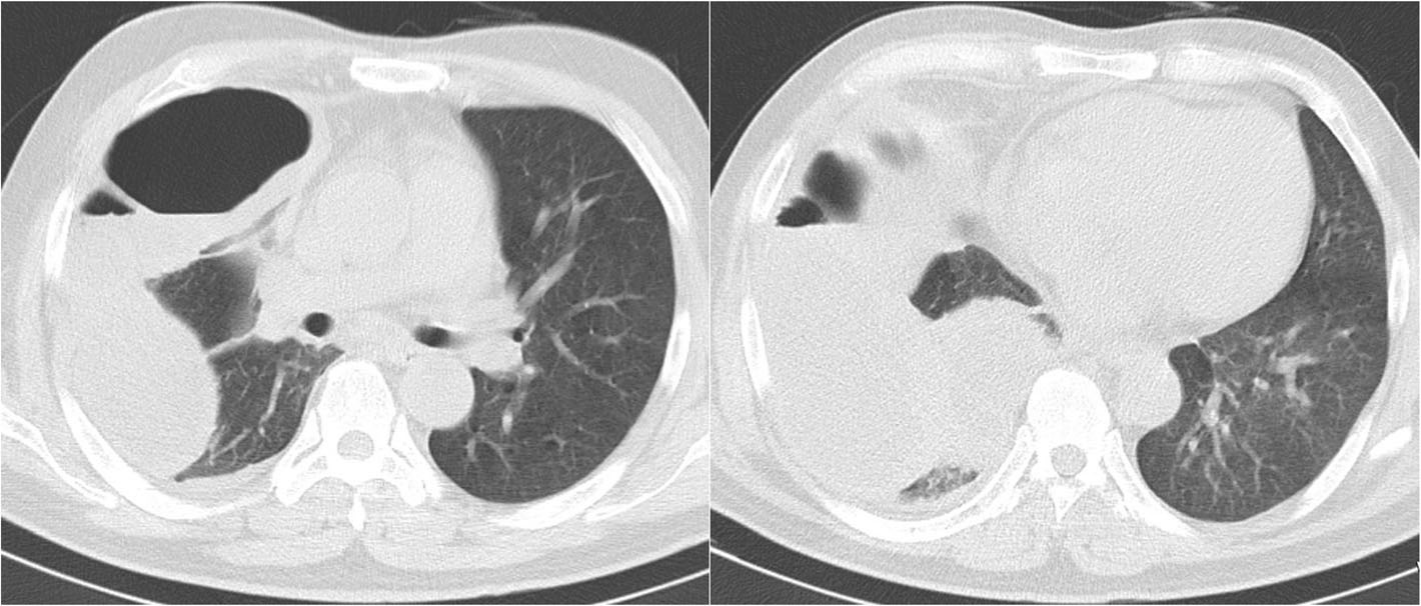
Chest computed tomography scan revealing right-sided massive pleural effusion with pneumothorax. Date: July 26, 2018
